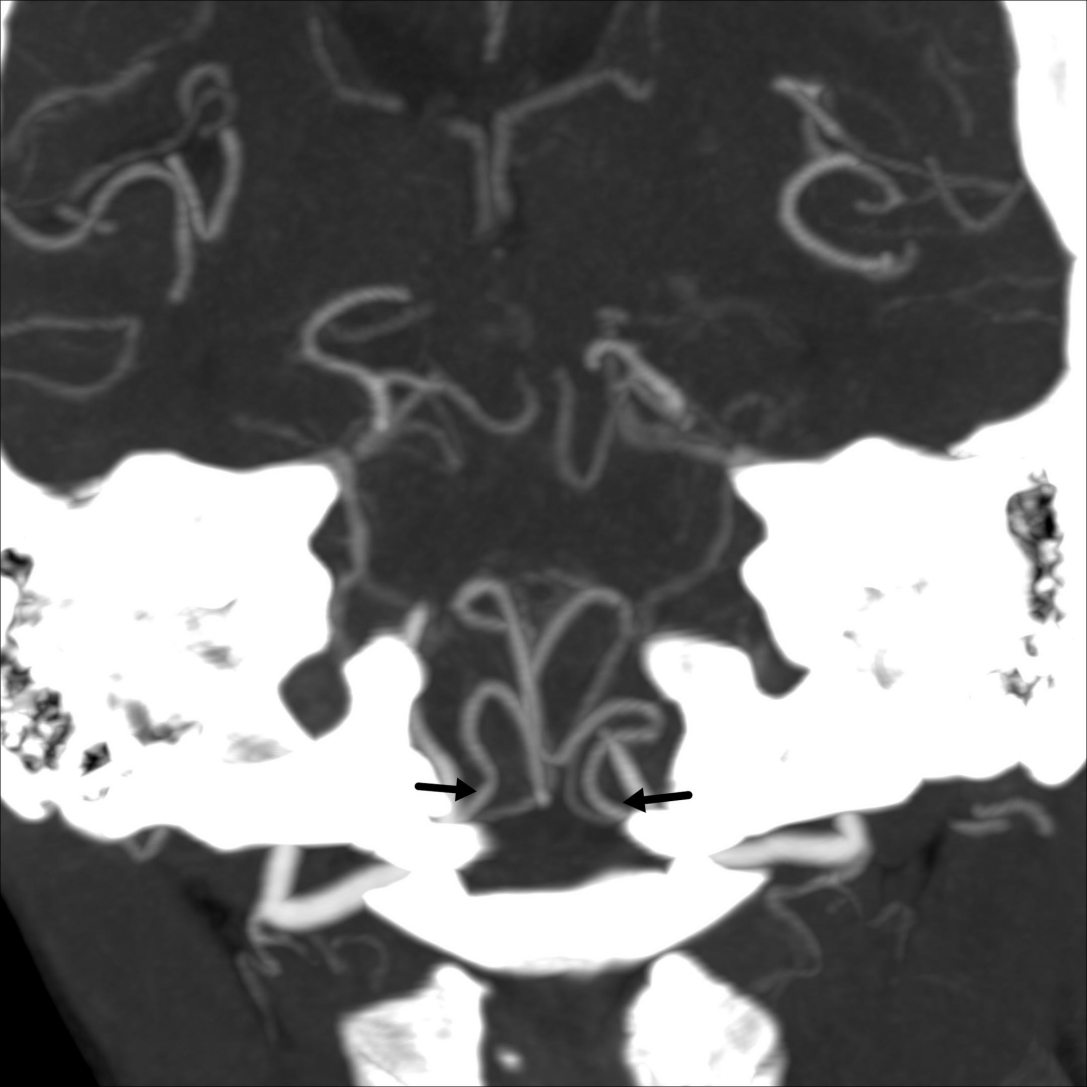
Computed tomography (CT) angiogram of the head Computed tomography angiogram of the head (maximal intensity projection images) coronal section showing patent bilateral posterior inferior cerebellar arteries arising from the intracranial vertebral arteries (black arrows).
